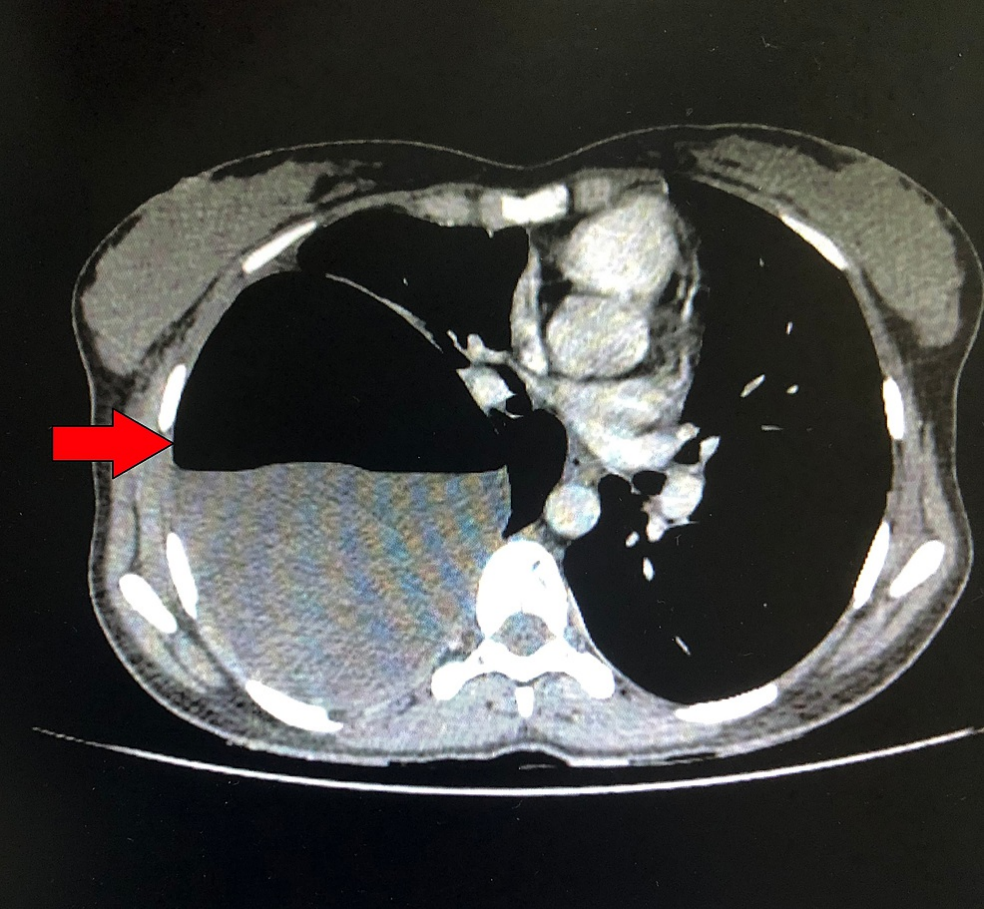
CT chest showing a well-defined fluid-filled cystic lesion in the right hemithorax CT- Computed Tomography
